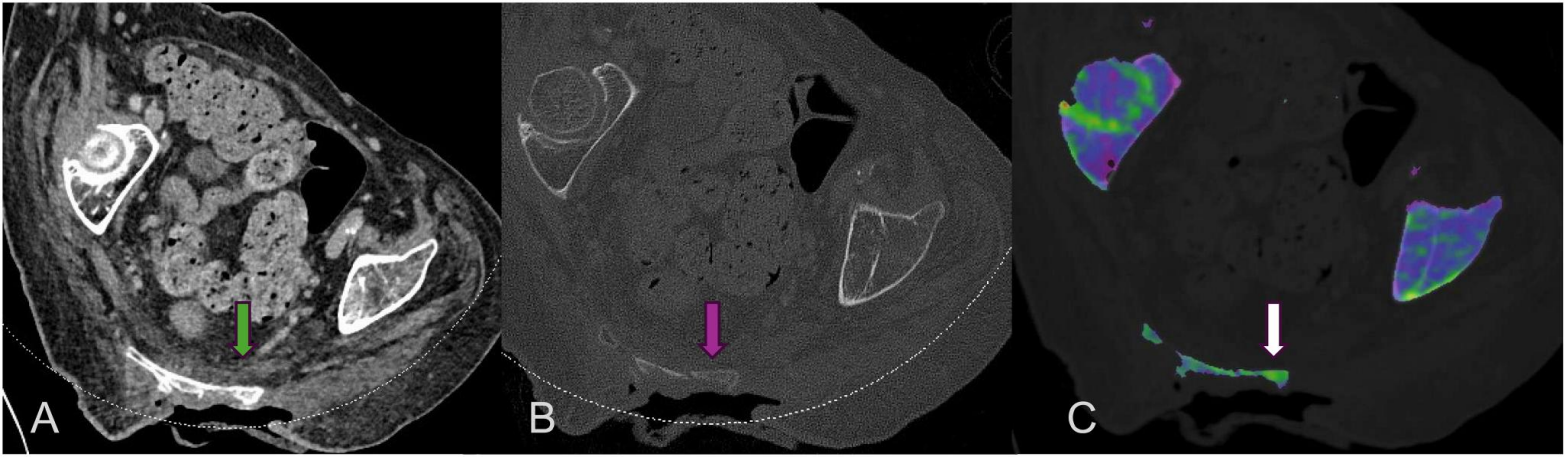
Dual energy CT of a patient was performed for clinical question of osteomyelitis. The patient had a sacral ulcer (Figure 2A & B). Dual energy maps confirmed the presence of marrow edema (Figure 2C) within the sacrum in keeping with osteomyelitis.
